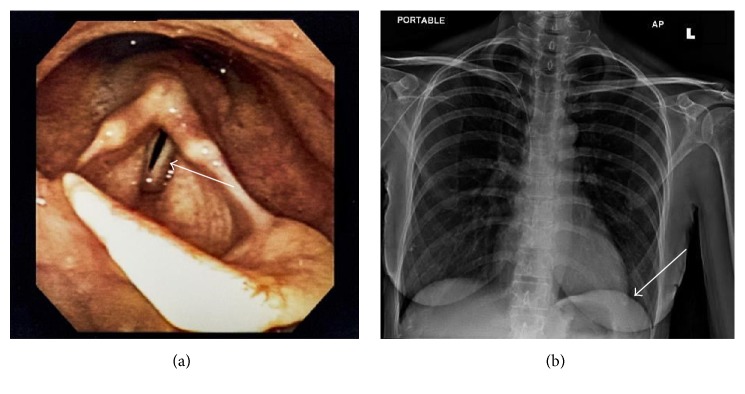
(a) Laryngoscopy photograph of the vocal folds, demonstrating left vocal fold paresis. (b) Portable chest radiograph with elevation of the left hemidiaphragm (arrow).
